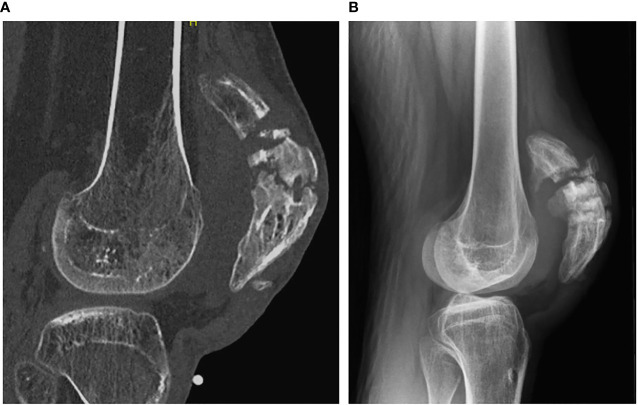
Sagittal CT view (A) and lateral radiograph (B) of the right patella showing the combination of the acute fracture and longstanding nonunion.
